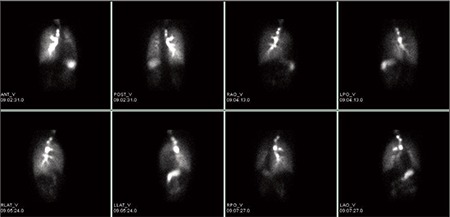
The plane fused SPECT/CT images of the abdomen show the activity in the ileal lumen
